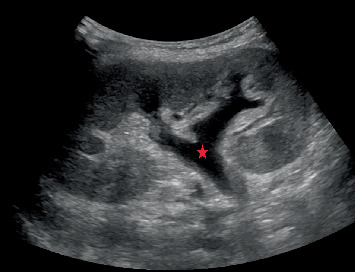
Transplant kidney ultrasound demonstrating hydronephrosis (red star).
